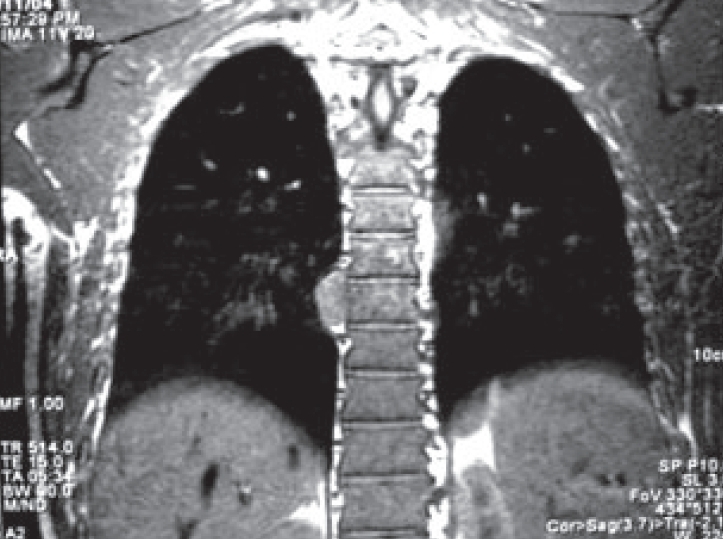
Sagital MRI depicting a right paraspinal mass in the mid thoracic spine
